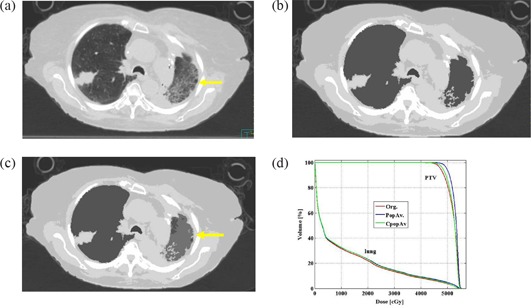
An example of a patient with pneumonia (yellow arrow) in the left lung. original treatment planning CT image (lung window); (b) treatment planning CT image of the same slice as 4a with the 5 bulk densities applied; (c) treatment planning CT image of the same slice as Figs. 4(a) and (b) where the individual average density is applied to the lung and $0.43\;g/{\text{cm}}^{3}$ is applied to the ground glass appearance area (yellow arrow); (d) DVH comparing the PTV and lung of the 3 plans.
